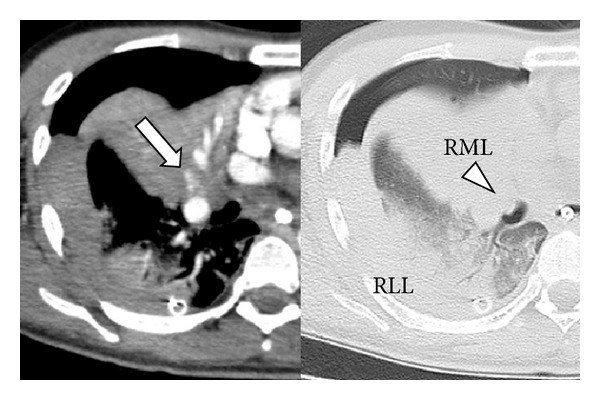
Chest computed tomography reveals extravasation of contrast medium in the right middle lobe (arrow) and compression of the middle lobe bronchus by a pulmonary hematoma (arrowhead). RML: right middle lobe; RLL: right lower lobe.
